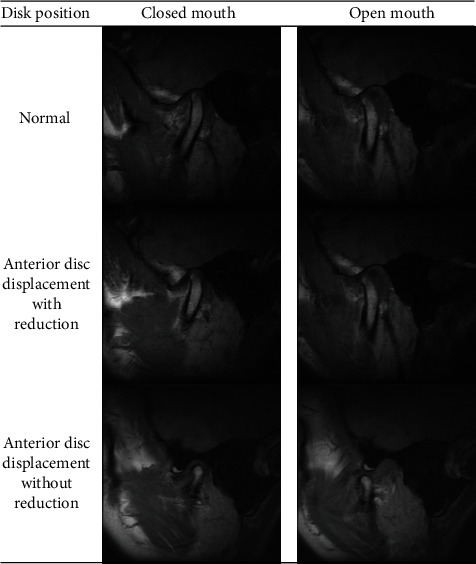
MRI images demonstrating normal and anterior disc locations with and without reduction.
